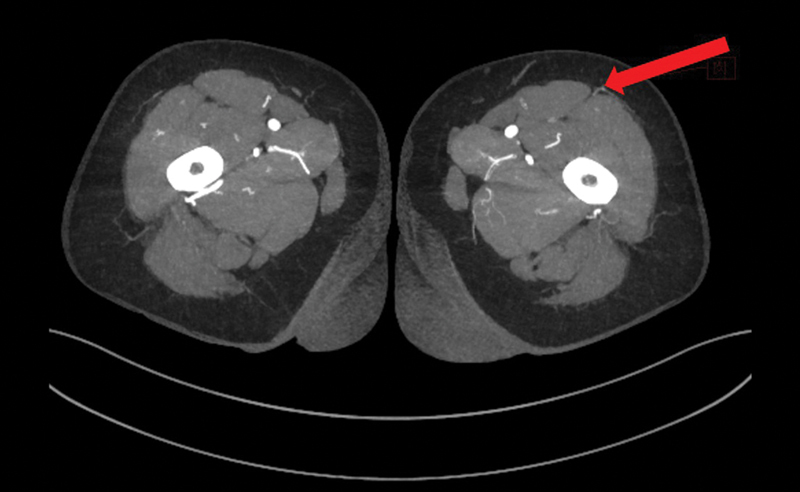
Computed tomography angiography. The red arrow shows a perforator of the descending branch of the lateral femoral circumflex artery.
